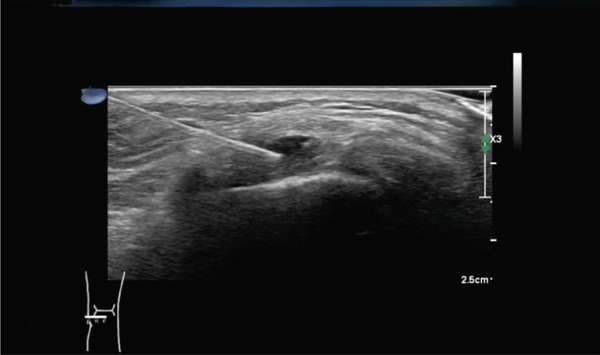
Ultrasound‐guided cortisone infiltration showing a multilobulated ganglion along the proximal course of the popliteus tendon, most likely corresponding to a tendon sheath ganglion.
